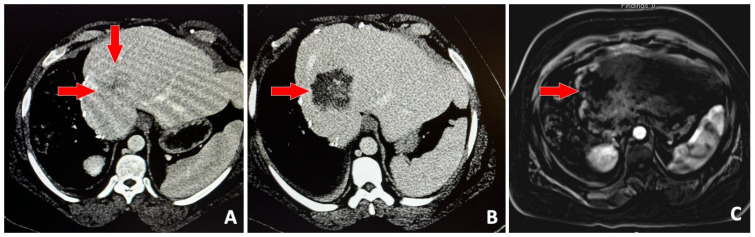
39-year-old male with history of right hepatectomy for metastatic colon cancer, presents with single hepatic metastasis and referred for histotripsy. Baseline contrast-enhanced CT (A) shows the 3.5 cm solid lesion (red arrows). Contrast-enhanced CT on post-procedure day #1 (B) shows no enhancement in the region that was targeted with histotripsy. Subtracted, contrast-enhanced T1-weighted MRI (C) 1-month post-procedure shows no evidence of viable tumor (red arrow).
